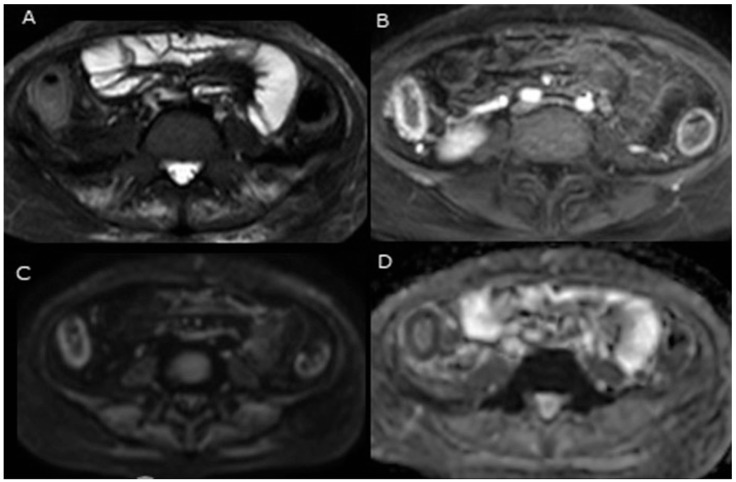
A Thirteen-year-old girl diagnosed with moderate activity of UC. On an axial T2-weighted image with fat saturation (A), noticeable findings included wall thickening measuring 7 mm and moderate hyperintensity (indicative of mural oedema) in the ascending and descending colon. An axial T1 post-contrast weighted image with fat saturation (B) exhibited pronounced mural enhancement. Axial DWI (C) and the corresponding ADC map (D) demonstrated conspicuous diffusion restriction within the colonic wall (figure taken from Figure 3 of Saleh et al. [60]).
